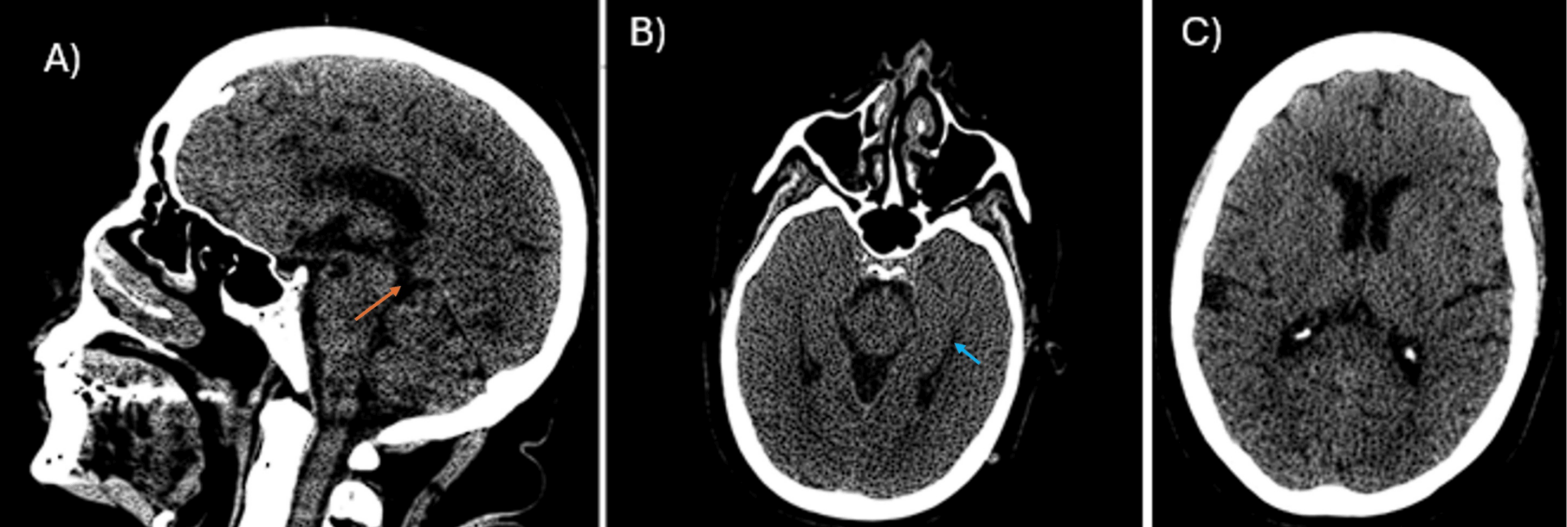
Non-contrast CT images. (A, B) Sagittal and axial non-contrast CT images acquired after the spontaneous resolution of the patient’s symptoms, showing complete resolution of the clot located within the mesencephalic aqueduct (orange arrow) and normalization of the size of the temporal horns of the lateral ventricles (blue arrow). (C) Axial non-contrast CT image acquired after the spontaneous resolution of symptoms, showing absence of transependymal edema and normal diameters of the bodies of the lateral ventricles.
